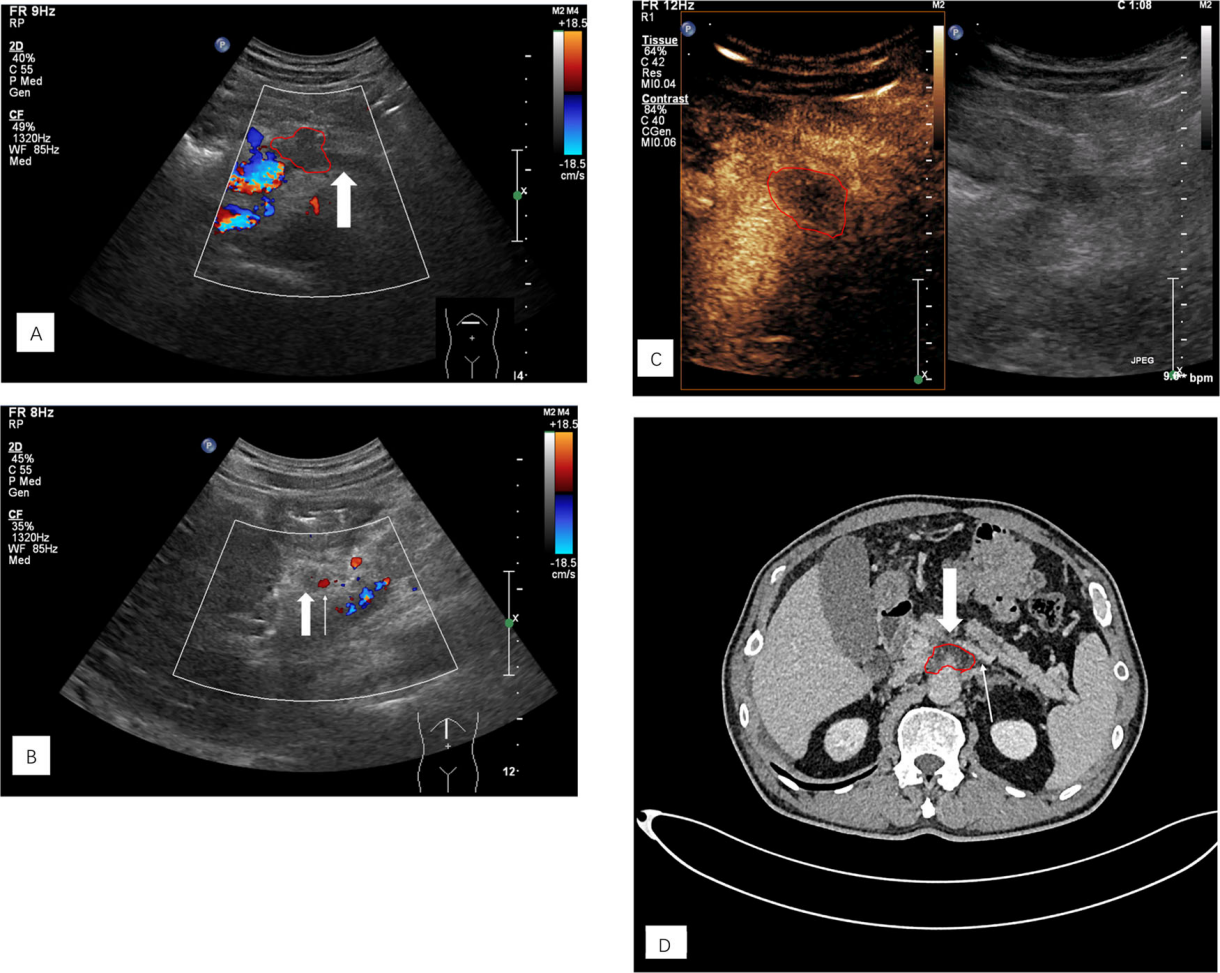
A 60-year-old male patient with PDAC located in the body of the pancreas. A The cross-sectional color Doppler scan showed that the lesion (red border) invaded the SPV, resulting in complete occlusion of the SPV (arrow). No blood flow signal was found on CDFI. B Longitudinal section of the color Doppler scan showed complete occlusion of the SPV (arrow), and no blood flow signal was observed on CDFI. The SPA could be seen beside the SPV (fine arrow). C On the venous phase CEUS scan, only the hypoenhanced lesion (red border) was visible, and the adjacent occluded SPV was difficult to distinguish. D On venous phase CECT scan, it could be seen that the SPV adjacent to the lesion (red border) was occluded (arrow), while the distal SPV was unobstructed (thin arrow)
